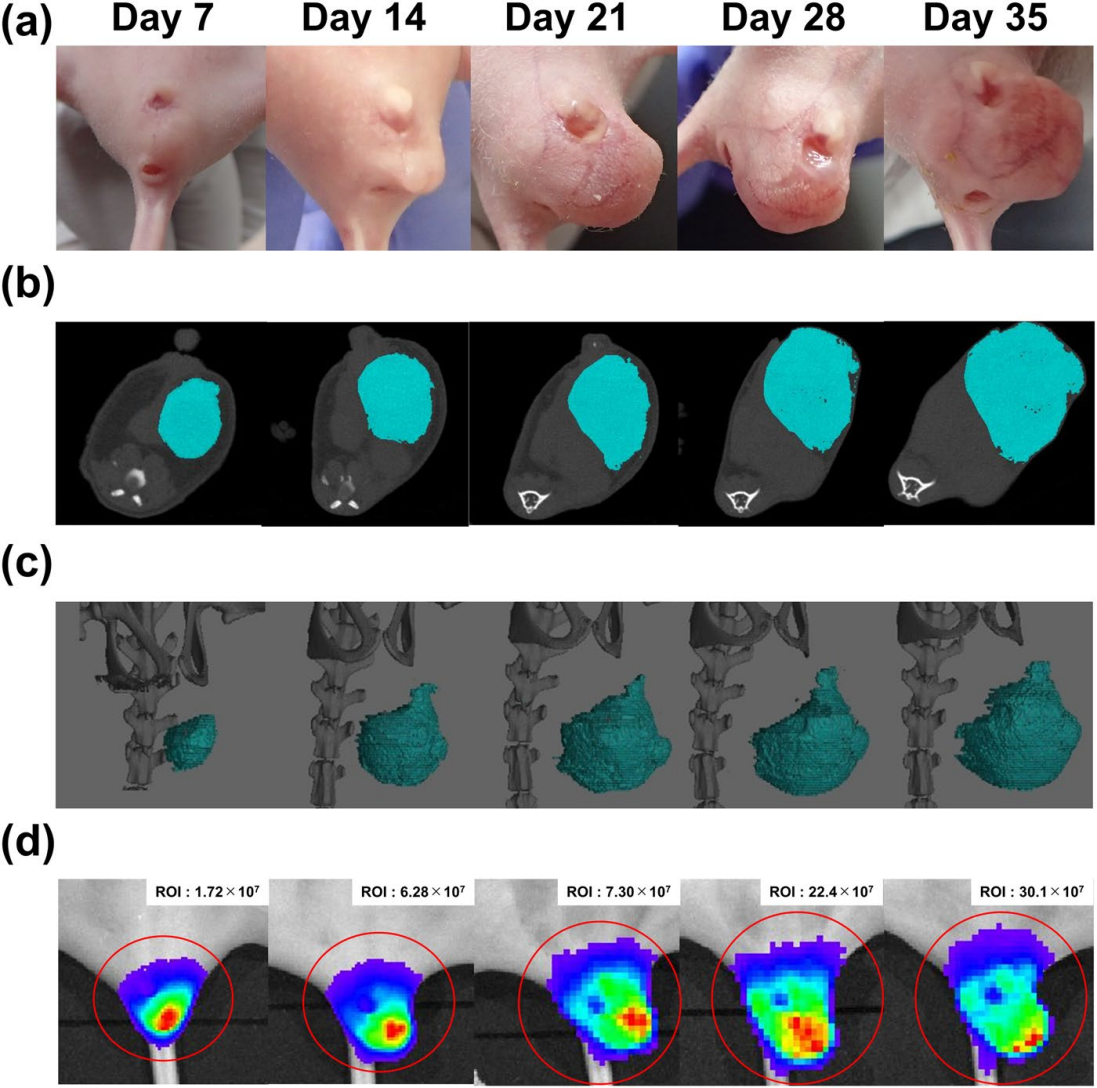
Representative images. (a) Images of tumors from the body surface. Images were taken every 7 days. (b) Images of tumors using the ROI contour tool with the Latheta CT scanner. (c) 3D images of tumors using VG Studio max 2.2. (d) Images from the Xenogen IVIS imaging system. (e) Total tumor volumes at 7, 14, 21, 28, 35, and 42 days after the inoculation of DLD-1 clone#1-Luc cells. (f) Total photon flux results within 6 min of the luciferin injection.
